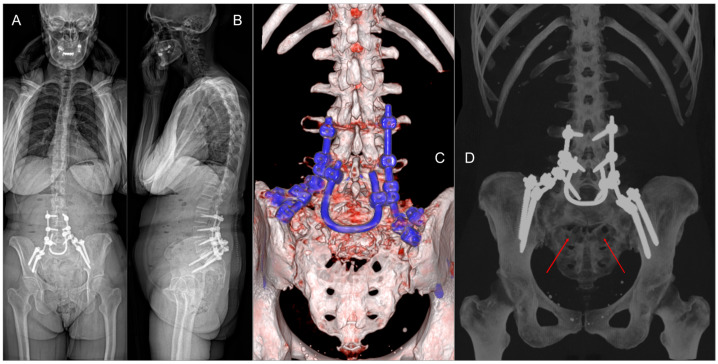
(A,B) Post-operative standing X-ray. (C,D) Six-month 3D CT scan reconstruction demonstrating the stability of the LPF and the residual sacral defect (red arrows).
